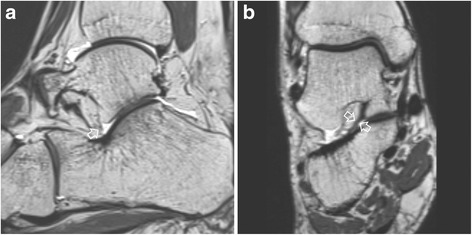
A 25-year-old women with subtalar instability. a Sagittal isotropic 3D T2-weighted image demonstrating the absence of anterior capsular ligament (open arrow). b On a coronal image along the posterior wall of sinus tarsi, the interosseous talocalcaneal ligament (open arrow) appears to be intact
